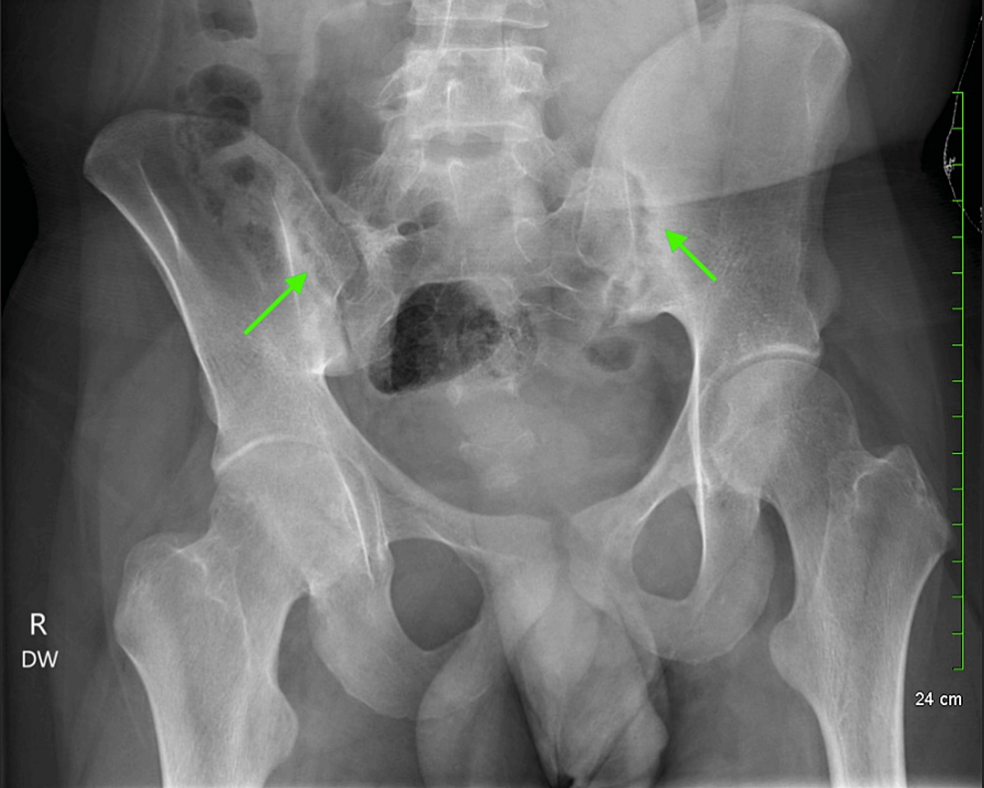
Bilateral hip and sacroiliac joint X-ray demonstrating joint space narrowing with sacroiliac joints showing subchondral lucencies with concern for bilateral inflammatory sacroiliitis.
